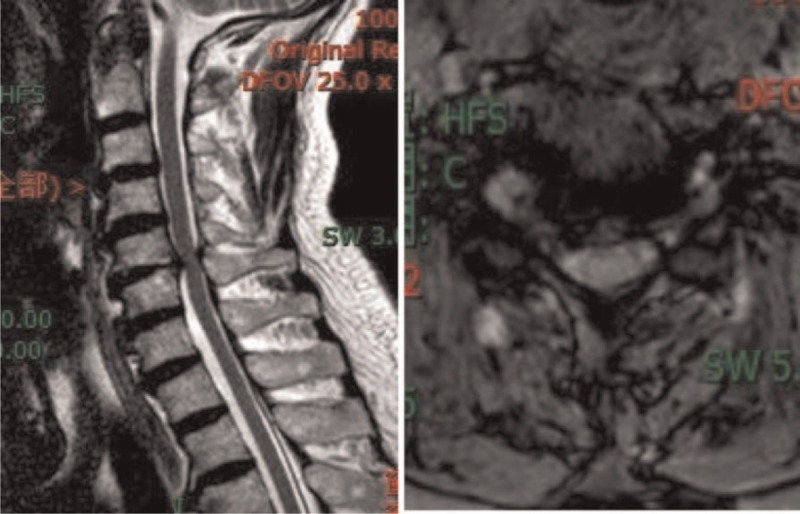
Axial (A) and sagittal (B) MRI showed C5/6 spinal cord compression. MRI = magnetic resonance imaging.
